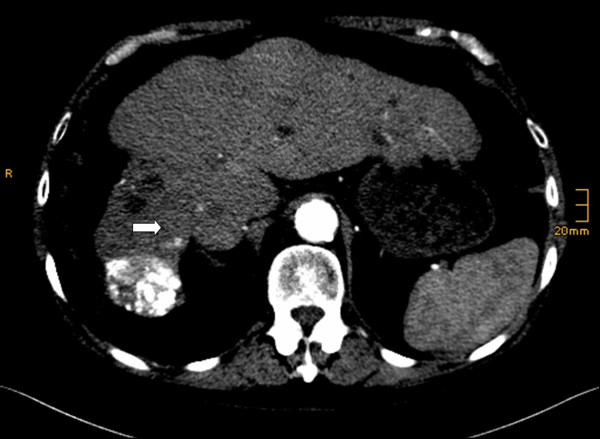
Control CT abdomen scan after eight weeks of AMT treatment. Early arterial examination time, showing liver cirrhosis after transarterial chemoembolization. TL1 disappeared.
